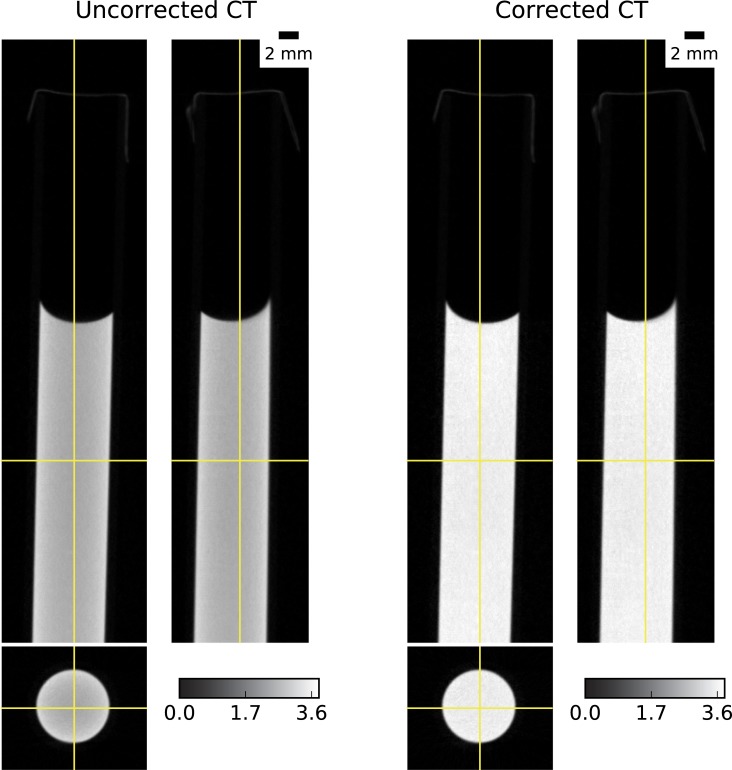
Uncorrected and corrected CT reconstruction of the water sample.
